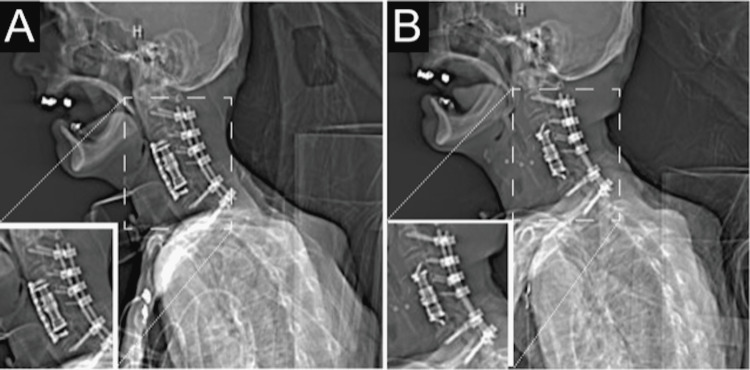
CT neck with contrast. Posterior fusion device with a facet and pedicle screws at C2-C5, C7, and T1 with anterior fusion plate and screw construct extending from the C3 level through the C6 level (Figure 3A). Post-surgical removal of the anterior fusion plate and screws (Figure 3B).
